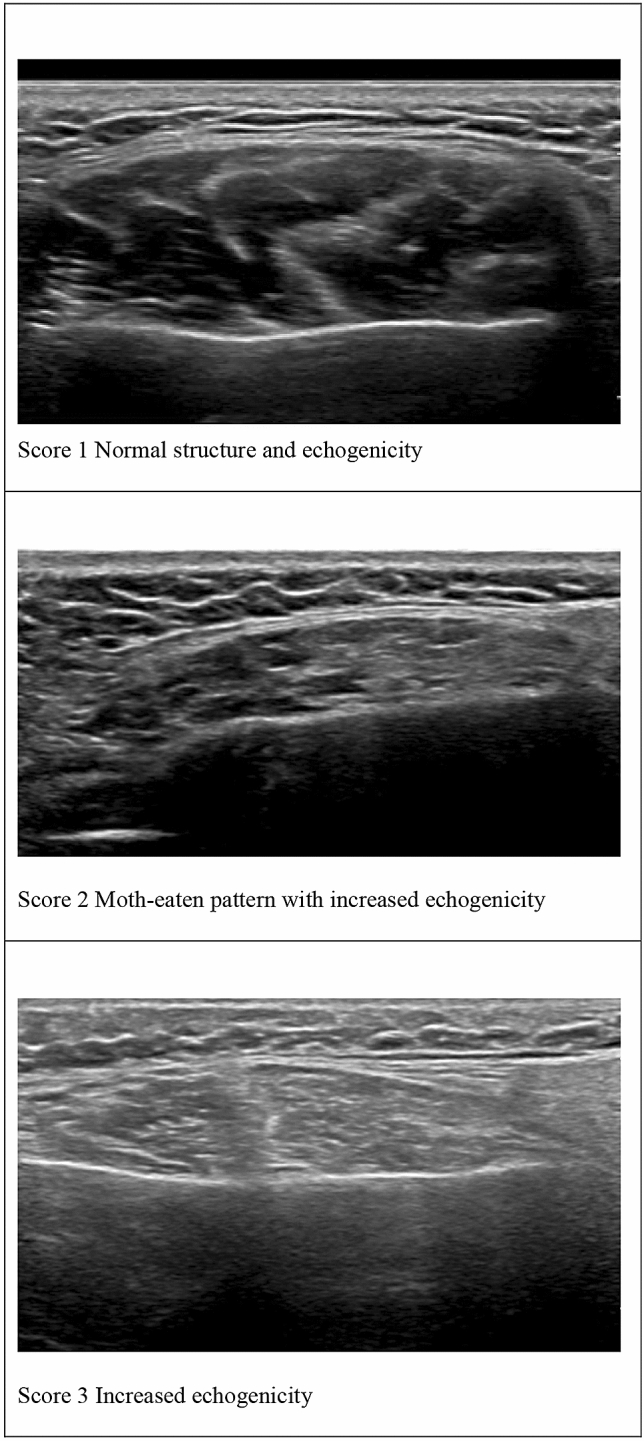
Muscle ultrasound images of the masseter muscle
